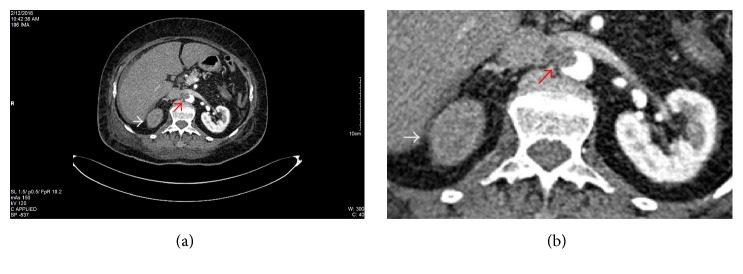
(a) Contrast-enhanced CT scan. Right renal artery occlusion with endoluminal aortic thrombus. The red arrow marks the endoluminal aortic thrombus at the emergence of the right renal artery. The white arrow shows the absence of nephrogram of the right kidney suggestive of renal infarction. (b) An enlarged image of (a) in which the red arrow marks the endoluminal aortic thrombus and the white arrow shows the right kidney with the absence of the nephrogram.
